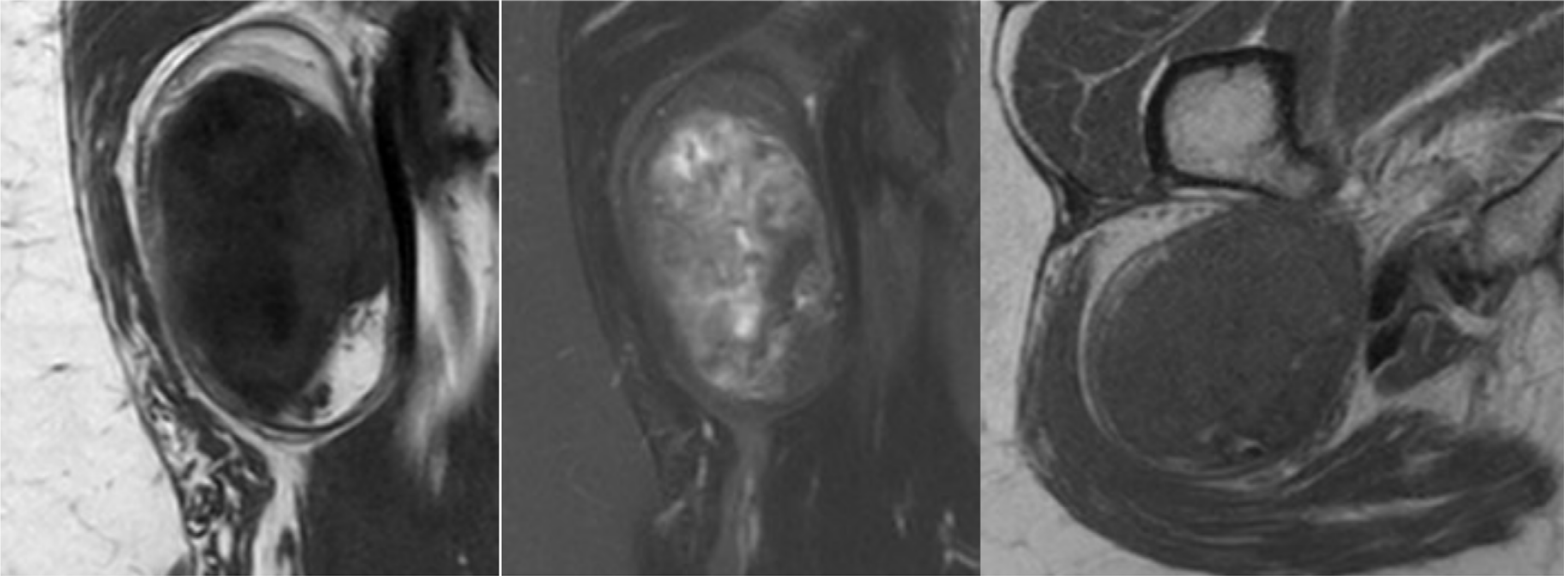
From left to right; front t1 and t2-weighted MRI sequences and axial t1 MRI sequence of the right hip.
